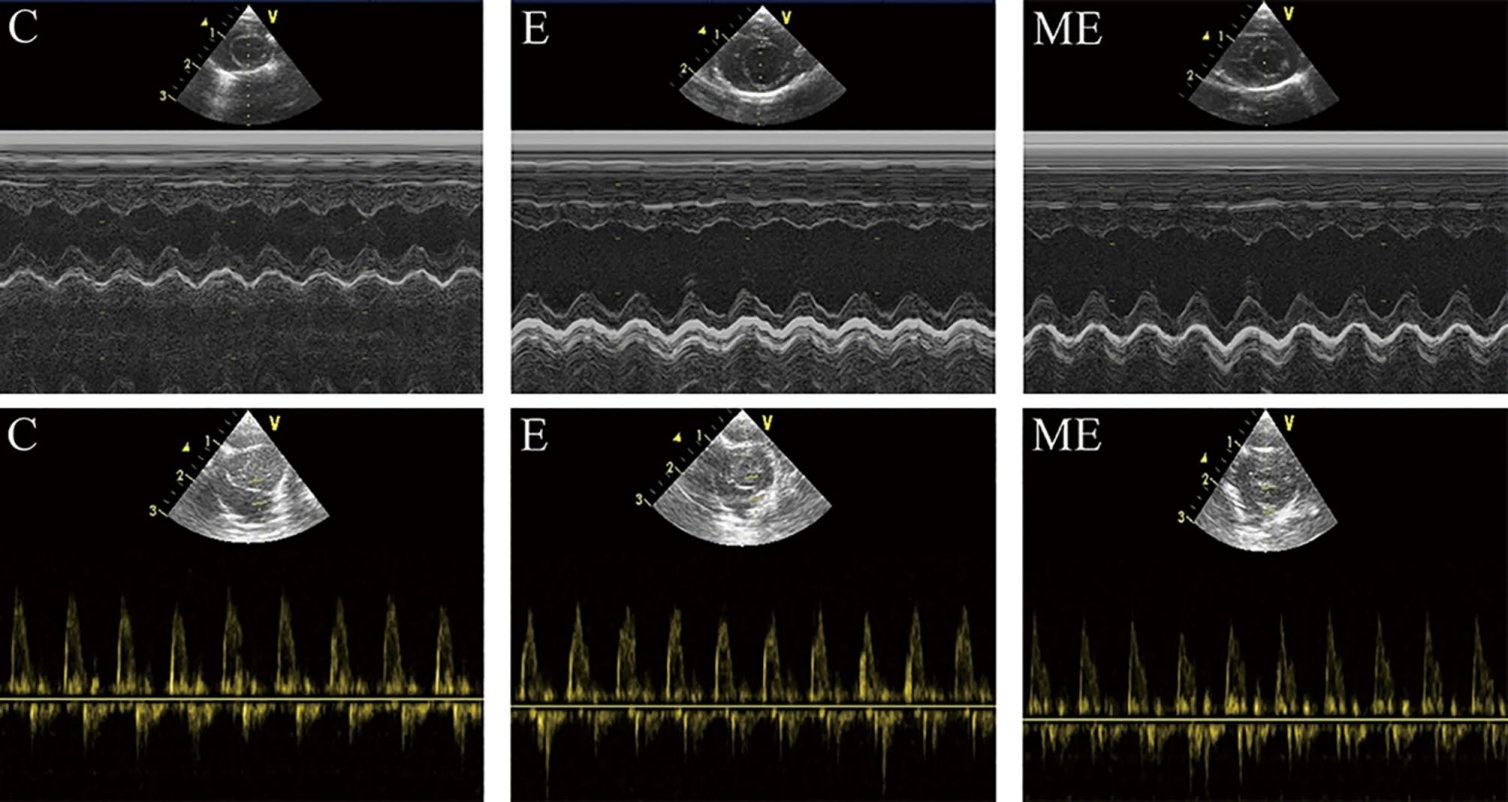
Changes of M-mode echocardiogram. The blood flow Doppler test records the ratio (E/A) of the peak blood flow velocity E in early diastole and the peak blood flow velocity A in late diastole.
